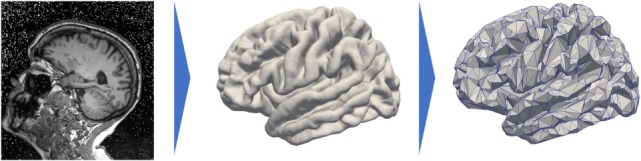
Data preprocessing that projects the cortical thickness of the raw MRI observation (left) on a mesh, namely, the FSAverage atlas constituted of 163.842 nodes per hemisphere (middle) before subsampling it and averaging the signal onto a 1,827-node graph (right).
